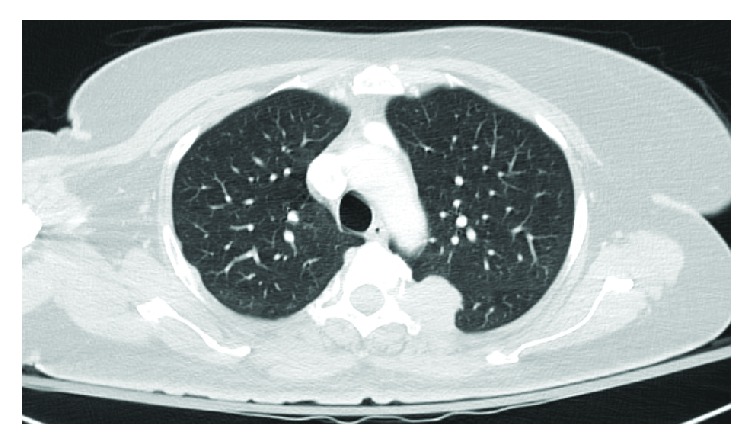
CT of the chest showing a subpleural mass in the upper left lobe that measured 3.3 × 3.0 × 2.7 cm concerning for metastasis.
